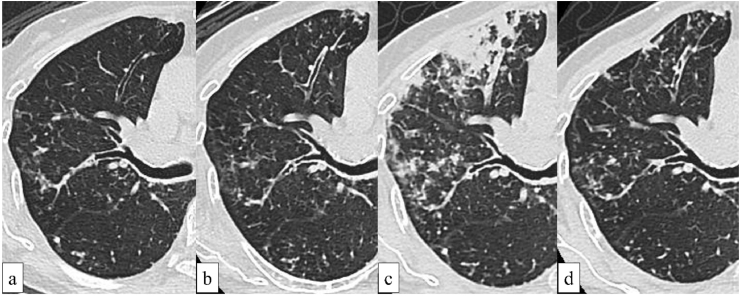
Chest computed tomography findings of the patient. a. Two years before admission, centrilobular nodules are observed in the right upper lobe. b. Five months before (two months after the discontinuation of the TNF inhibitor) admission, the centrilobular nodules have extended to the S3 region. c. On admission, the centrilobular nodules have increased in number and enlarged rapidly over five months. Furthermore, the lesions have aggregated and exhibit consolidation. d. Two months after starting chemotherapy, the consolidation has disappeared.
